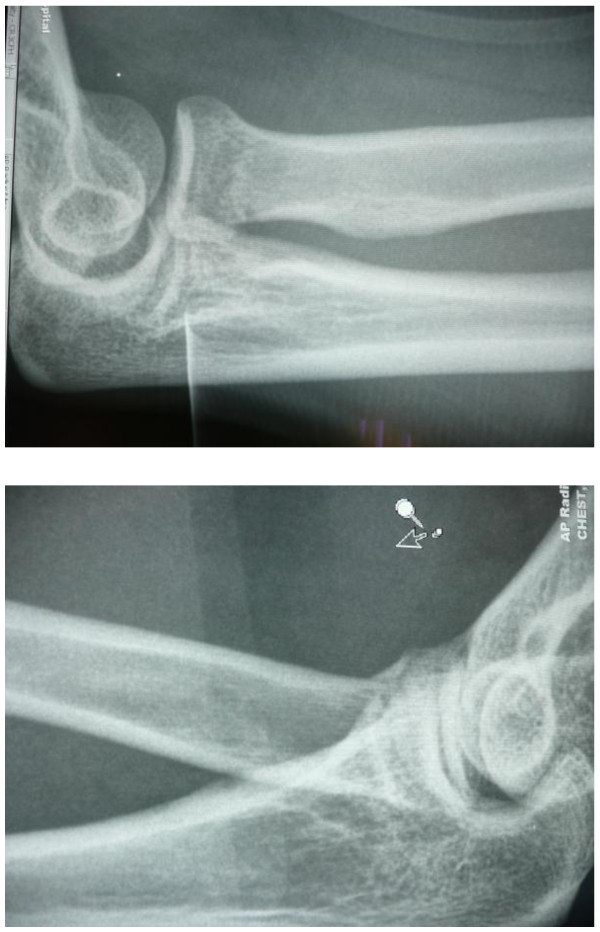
Case pair B, two radiographs of the elbow of two injured passengers of the same armored personnel carrier after having hit an improvised explosive device (IED), showing contralateral fractures of the radial neck.
